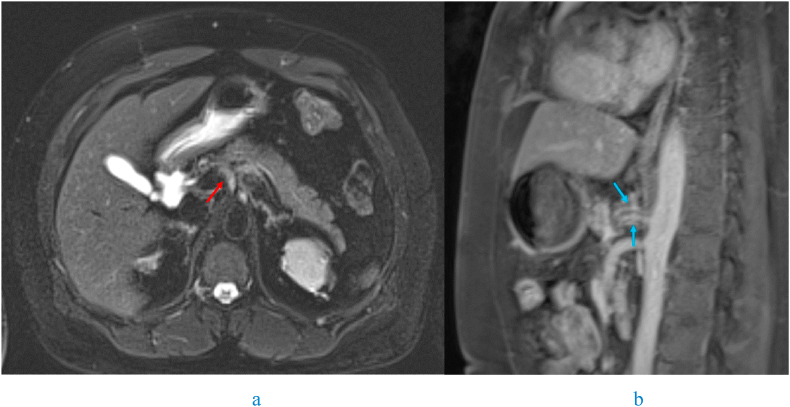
a and bFat-saturated axial T2-weighted image showing the increased signal intensity of the celiac artery wall (red arrow) compatible with oedema and inflammation (figure a). Sagittal T1-weighted fat-saturated gadolinium-enhanced MR image showing circumferential thickening and increased mural enhancement of the wall of the celiac artery (blue arrows) in addition to luminal narrowing (figure b).
